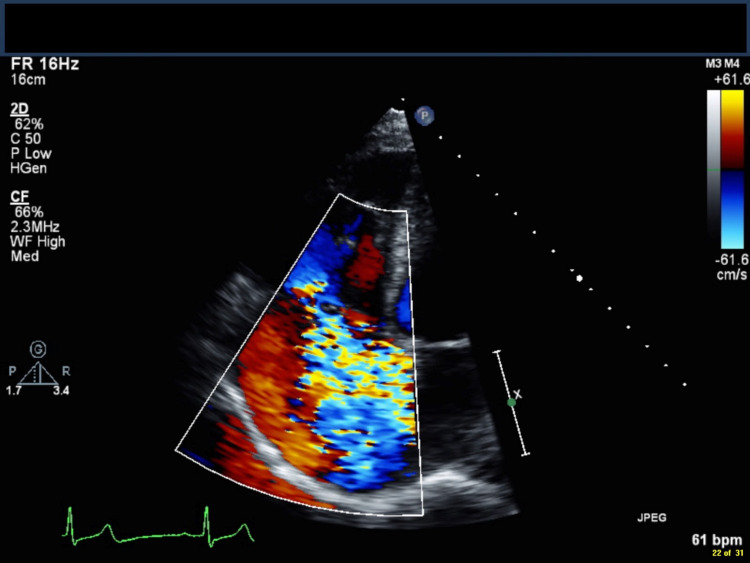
A transthoracic echocardiogram in apical four-chamber view showing severe, torrential central tricuspid regurgitation (blue jet).
